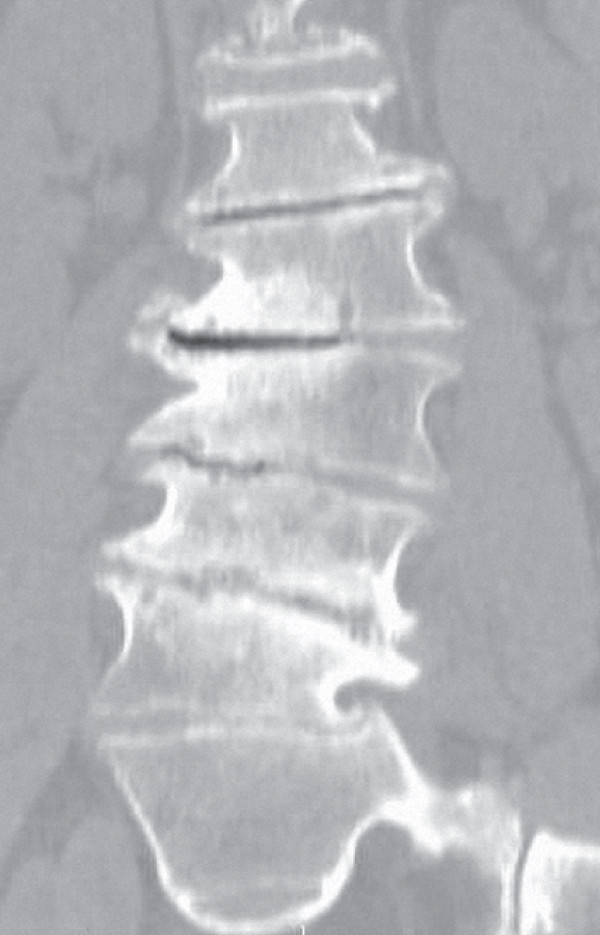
Pre-operative coronal computed tomography (CT) image of our patient, a 70-year-old man with marked thoracolumbar scoliosis, degenerative osteoarthritic changes characterized by high-grade osteophytes, endplate sclerosis and significant inter-vertebral disc space collapse.
